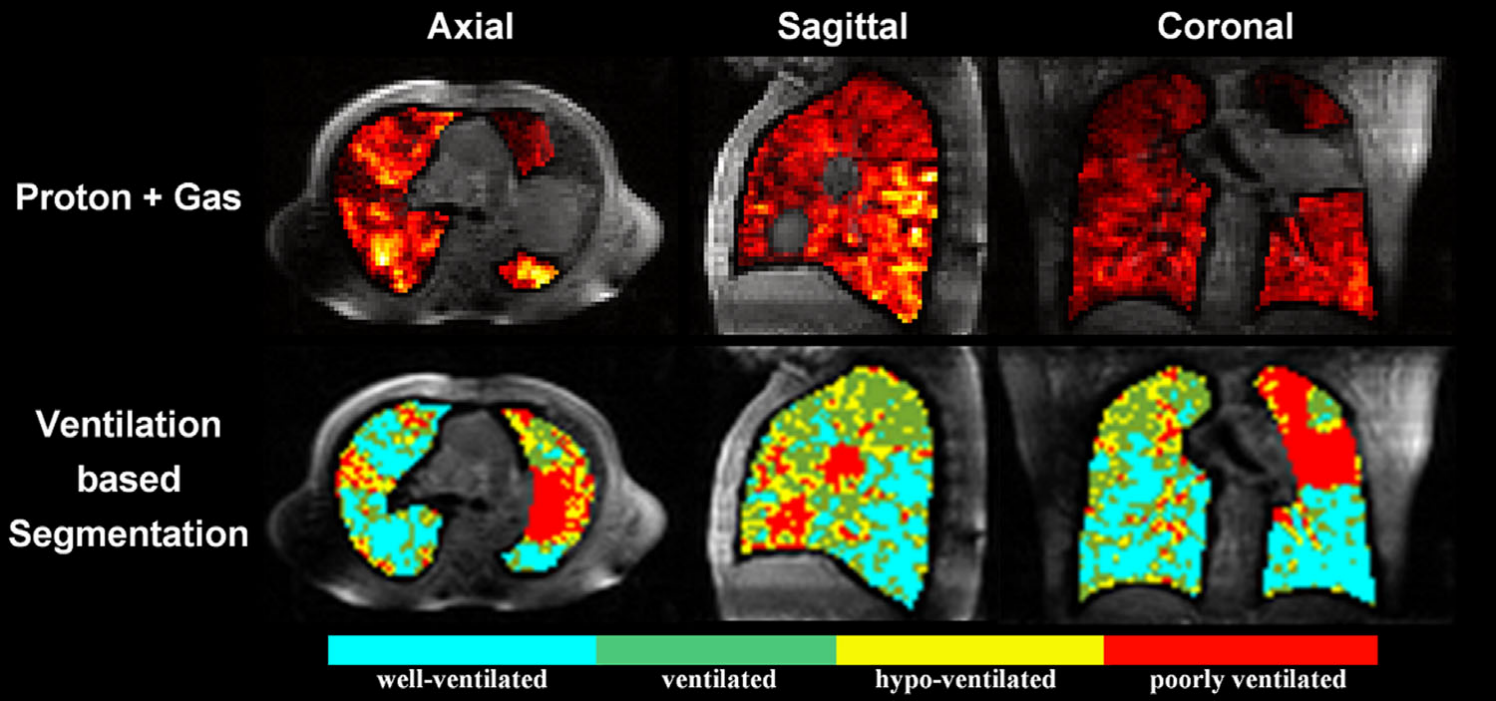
Upper row: HP xenon‐129 gas MR images (color) overlaid on proton MR images (grayscale) from patient P1 (Age 60, Male, T3N3M0, Stage III C, AJCC Version 8). Lower row: Segmentation of lung into four clusters based on the signal intensity of HP xenon‐129 gas MR images. The well‐ventilated, ventilated, hypo‐ventilated and poorly ventilated areas are shown in cyan, green, yellow, and red colors, respectively
